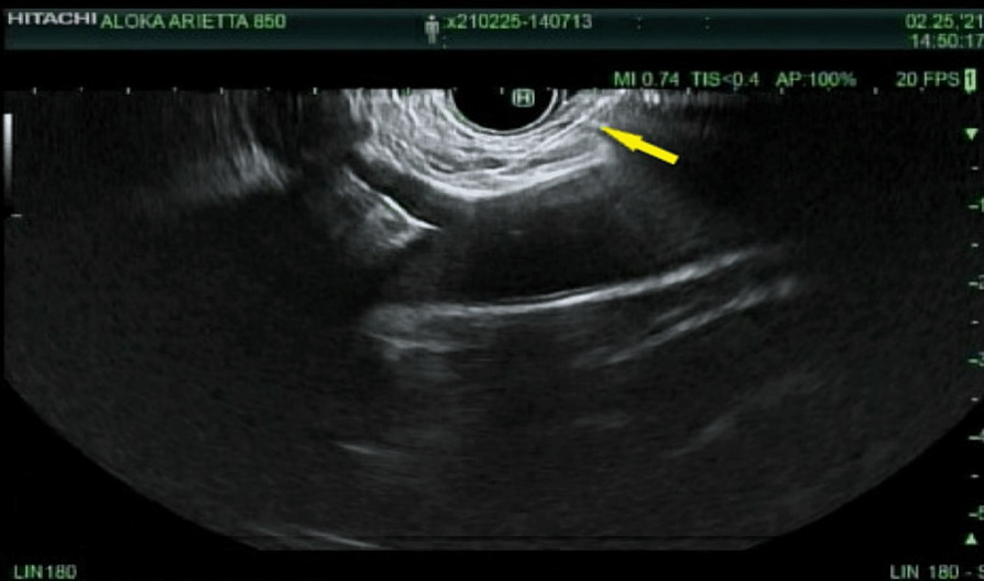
EUS-guided celiac plexus nerve block. Shown is the EUS-guided celiac plexus nerve block. The arrow indicates a 22-gauge needle inserted into the celiac ganglia. EUS: endoscopic ultrasound.
